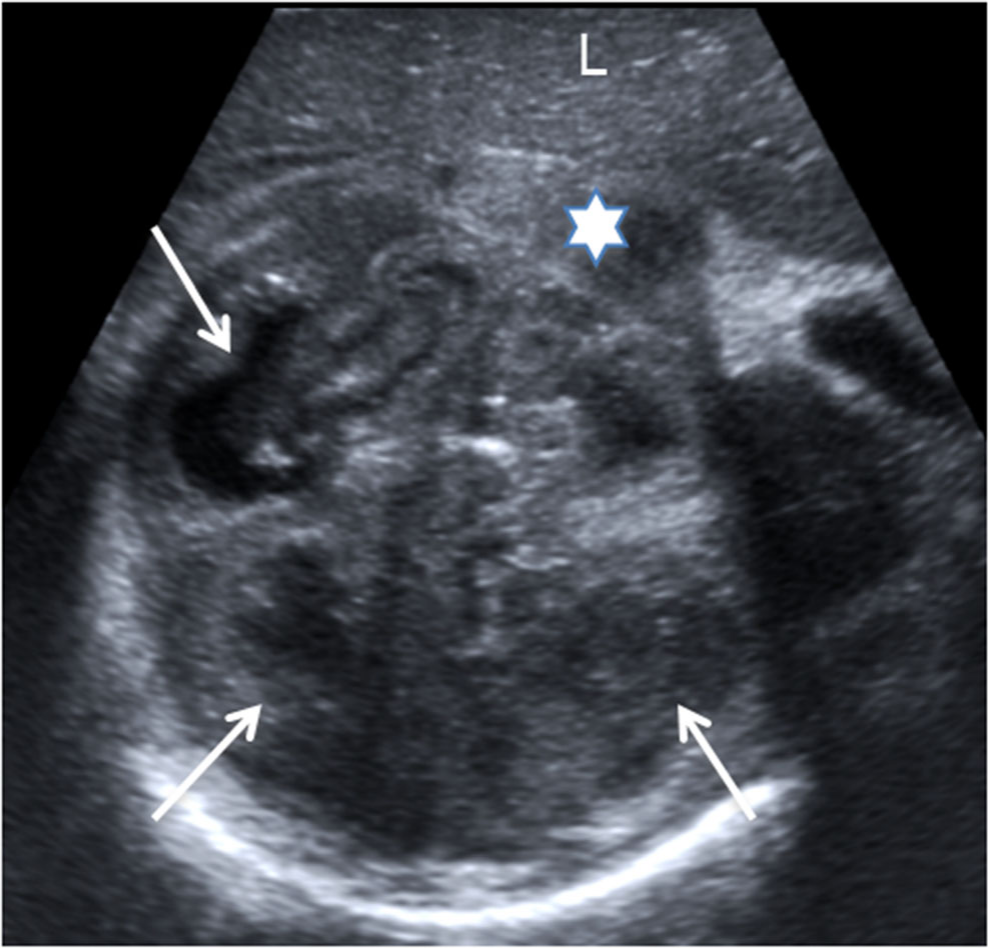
Diffuse XPN. Transverse sonogram image shows the right kidney parenchyma replaced with multiple hypoechoic masses (*white arrows*). Perinephric fluid collection is present (*star*). Liver (*L*)
